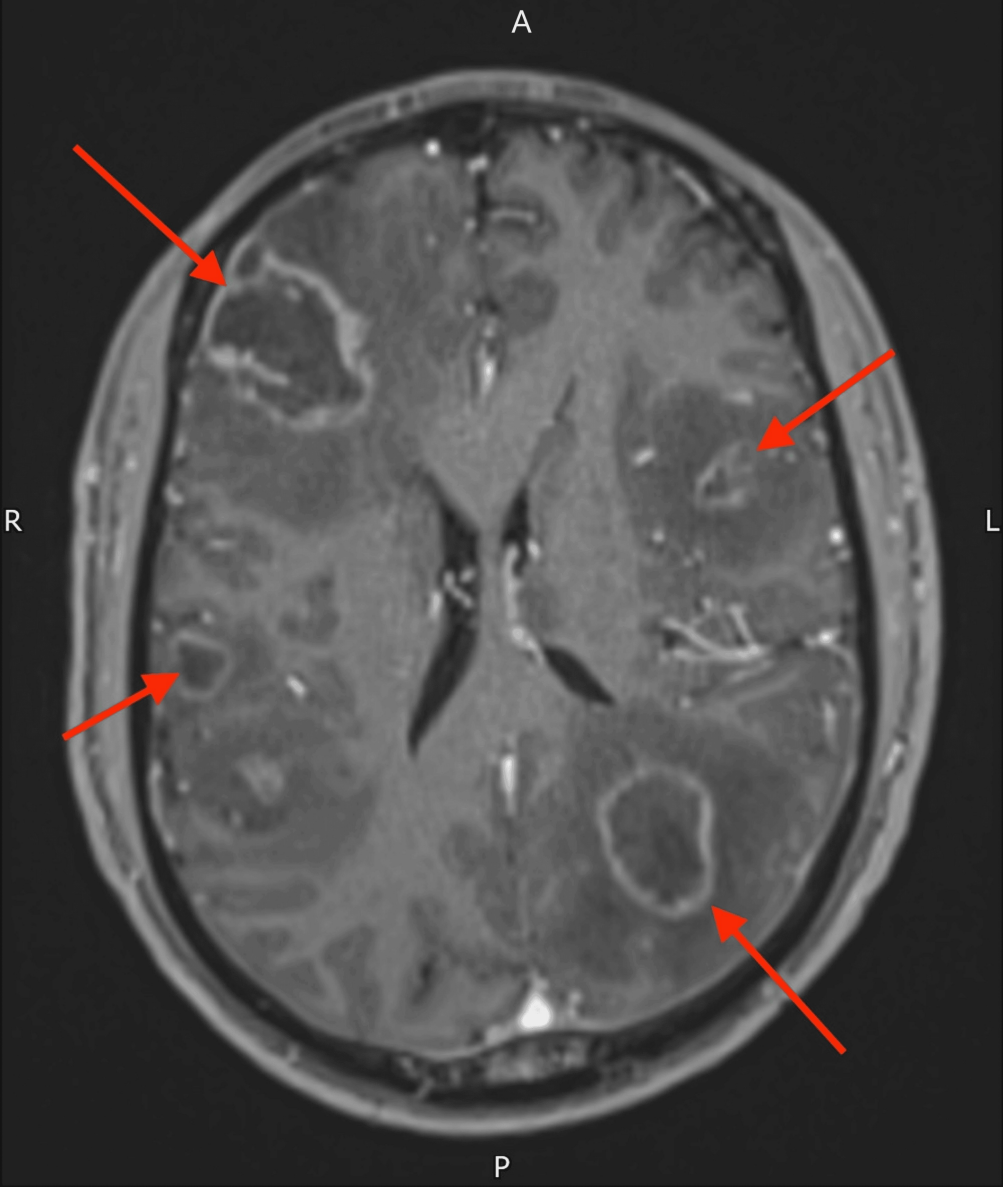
MRI of the head with contrast showing irregular ring-enhancing lesions in both cerebral hemispheres with surrounding vasogenic oedema MRI: magnetic resonance imaging
